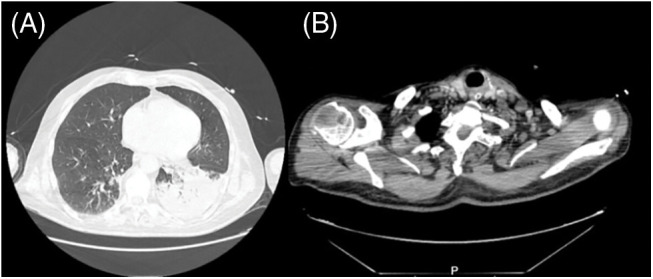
Thoracicabdominopelvic CT scan revealed extensive consolidation to the lower lobe of the left lung with foci of necrosis (A) and presumably metastatic lesions to lymph nodes, peritoneal cavity and bones (B).
